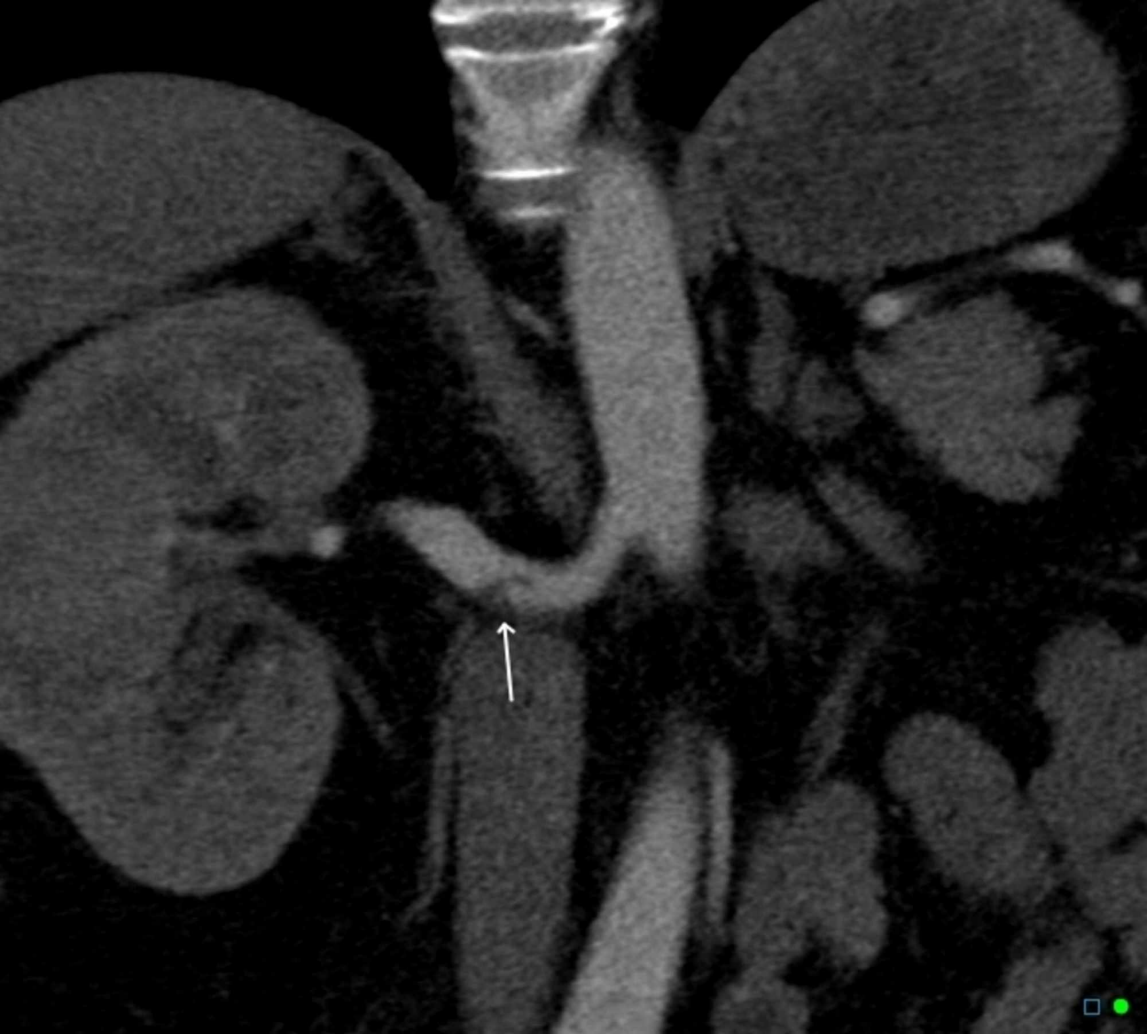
Coronal view of computed tomography angiography (CTA) showing right renal artery dissection - white arrow Figure courtesy: Dr Chris O'Donnell; https://radiopaedia.org/; from the case https://radiopaedia.org/cases/41977
